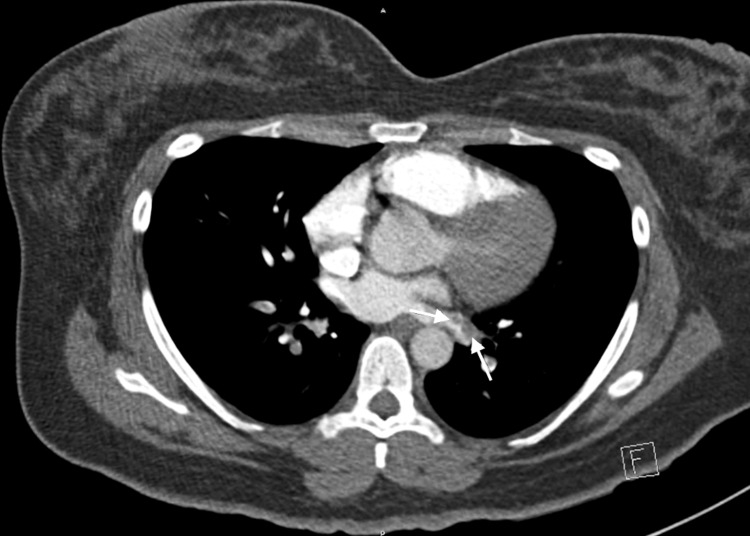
Axial image of the CTA of the chest with pulmonary vein thrombosis (arrows)
